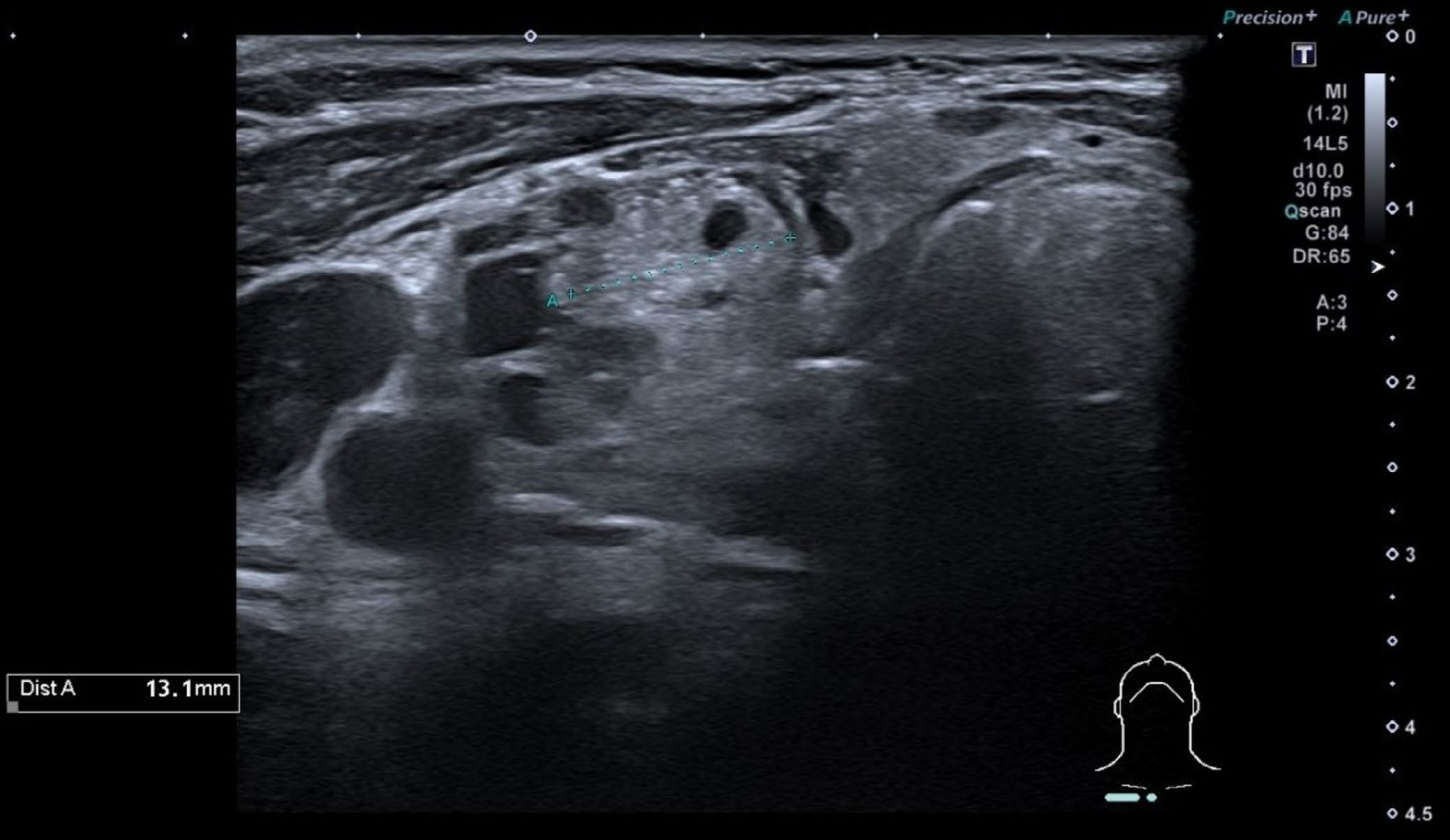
Thyroid ultrasound (transverse view); an isoechogenic solid nodule of 13 mm (EU‐TIRADS 3).
